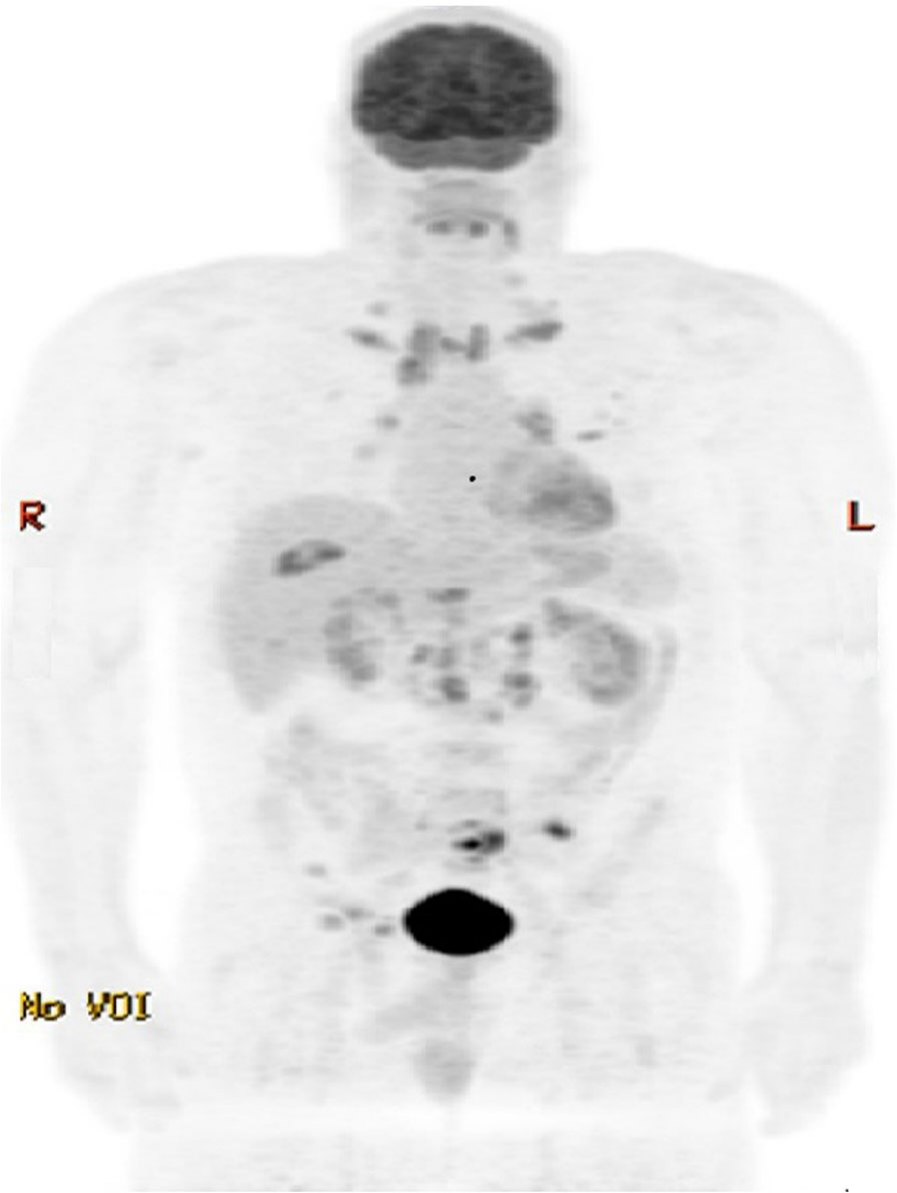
Nuclear positron emission tomography-computed tomography showing multiple foci involving lymph nodes at the paratracheal, left supraclavicular, para-aortic, and right inguinal regions. There was also hypermetabolic activity involving bilateral lung parenchyma and left tenth rib
